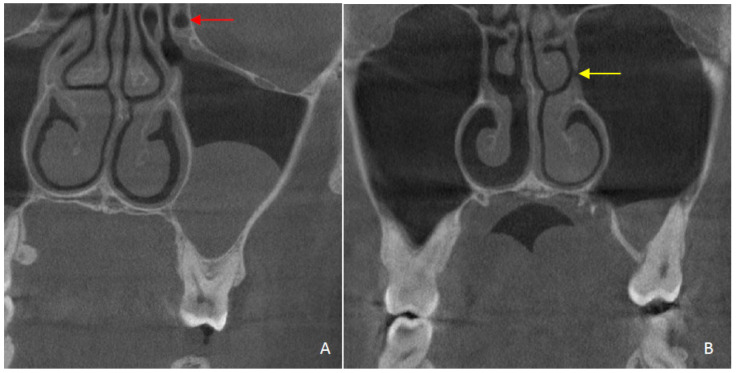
Coronal section of a CBCT scan is presented. (A) A Haller cell (red arrow) and a maxillary sinus pathology are observed; (B) the AMO (yellow arrow) and a maxillary sinus pathology are observed.
